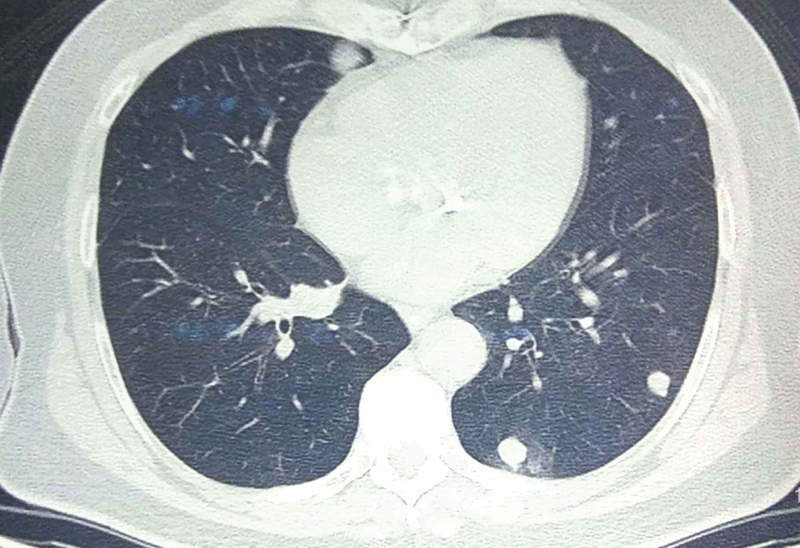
Positron emission tomography–computed tomography scan thorax shows lesions in left lung field.
